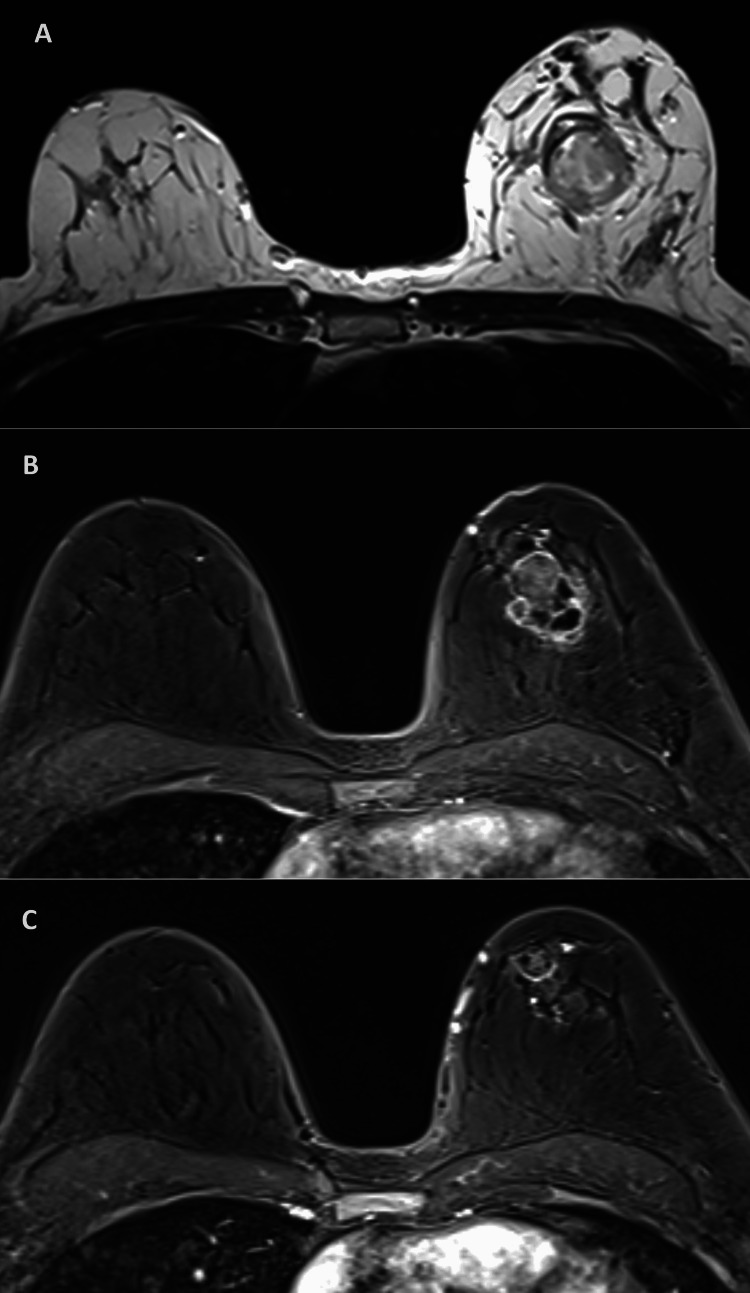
Breast MRI (A) Before neoadjuvant chemotherapy. (B) After neoadjuvant chemotherapy: some necrosis is visible indicating a response to treatment, but also present anterior and medial proliferation. (C) After neoadjuvant chemotherapy: new lesion located above the original tumor indicating progression
